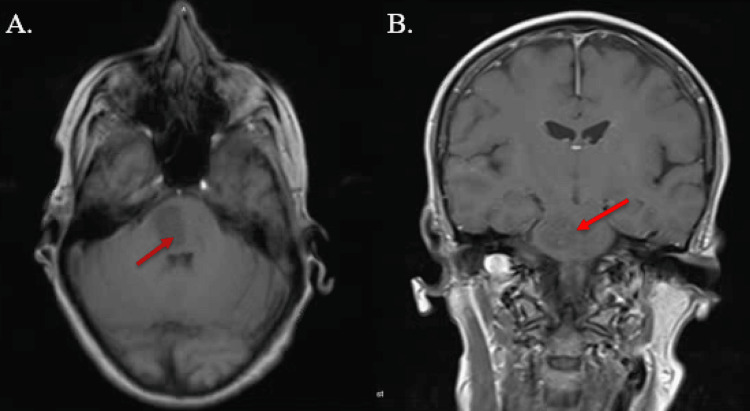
MRI axial and coronal views, respectively, showing intraparenchymal hemorrhage at the level of the pons measuring 2.7 x 1.8 cm with significant edema at about 18 hours after presentation in the ED. The patient had signs of significant left-sided hemiparesis, left-sided facial droop, fixed leftward gaze, and slurred speech at that time.
